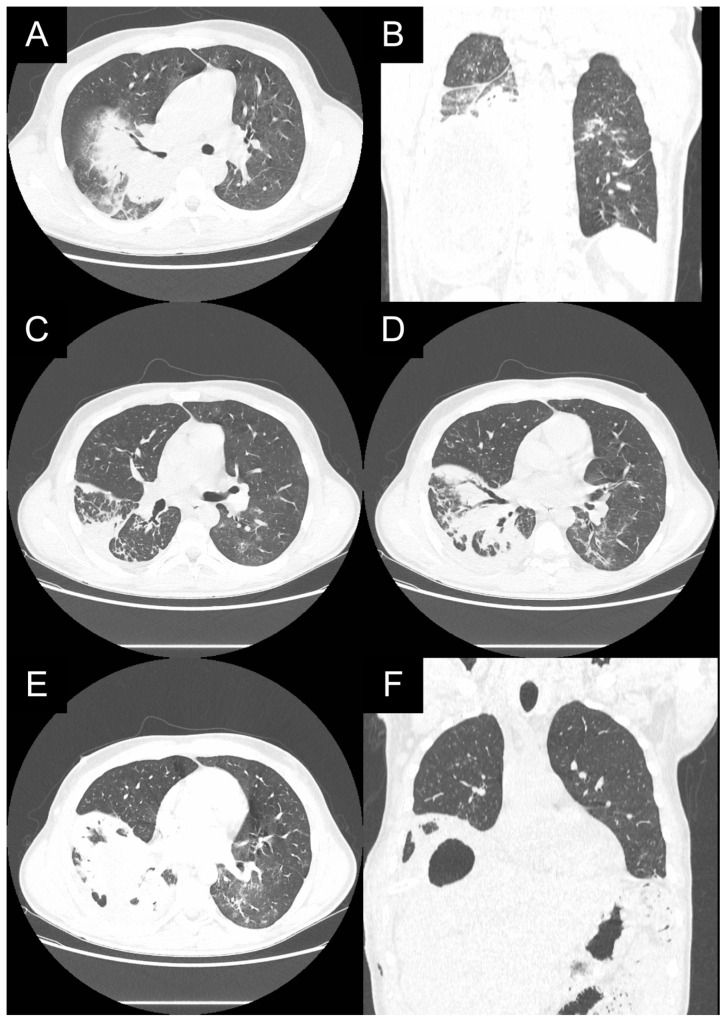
(A–F): A chest CT of patient 1 showing ruptured continuity of the hepatic lesion. The right lower lobe bronchi are filled with fluid from hepatic lesion. At bronchoscopy, an S10 fistula was detected.
